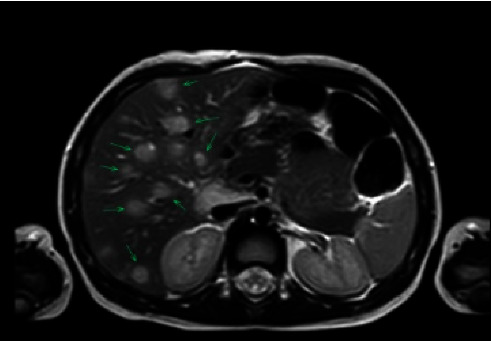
MRI pelvis. Arrows pointing to innumerable small hepatic ring enhancing lesions.
